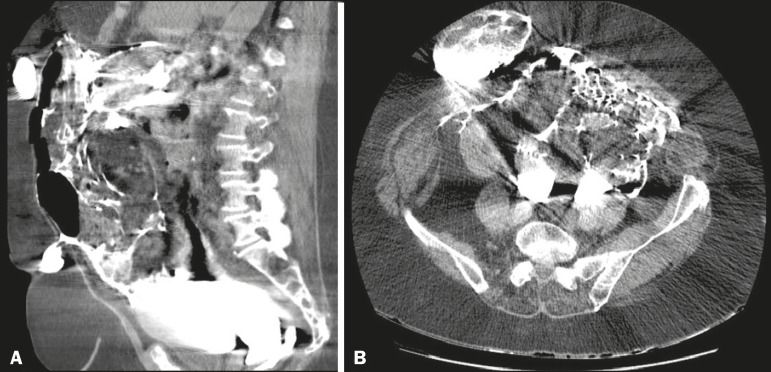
Computed tomography scans of the abdomen, in the axial (A) and sagittal (B)
